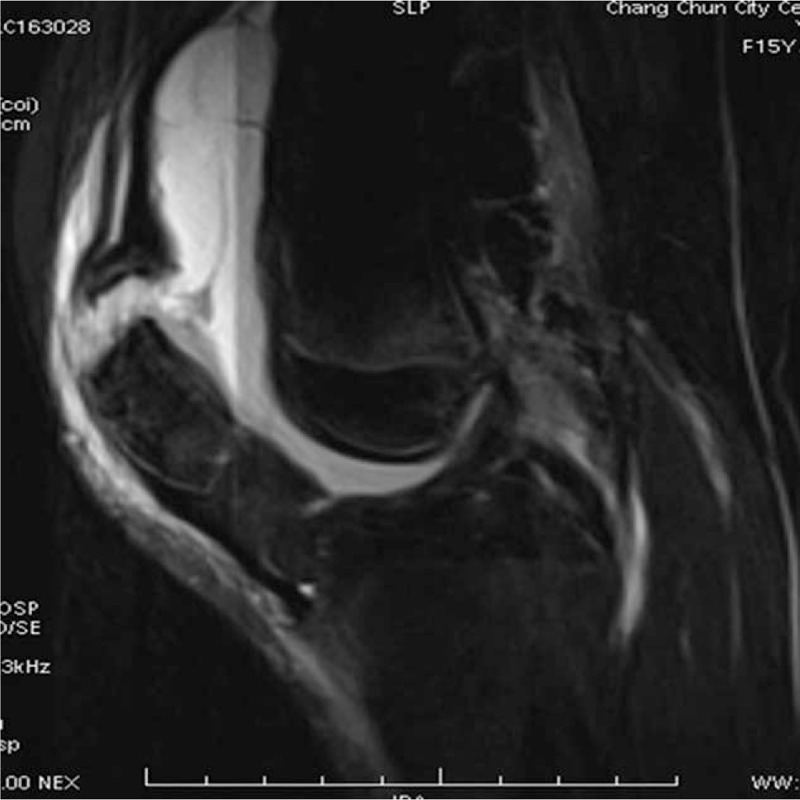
MRI sagittal T2 with fat suppression showed a superior pole fracture of the patella with an intact quadriceps tendon. MRI = magnetic resonance imaging.
